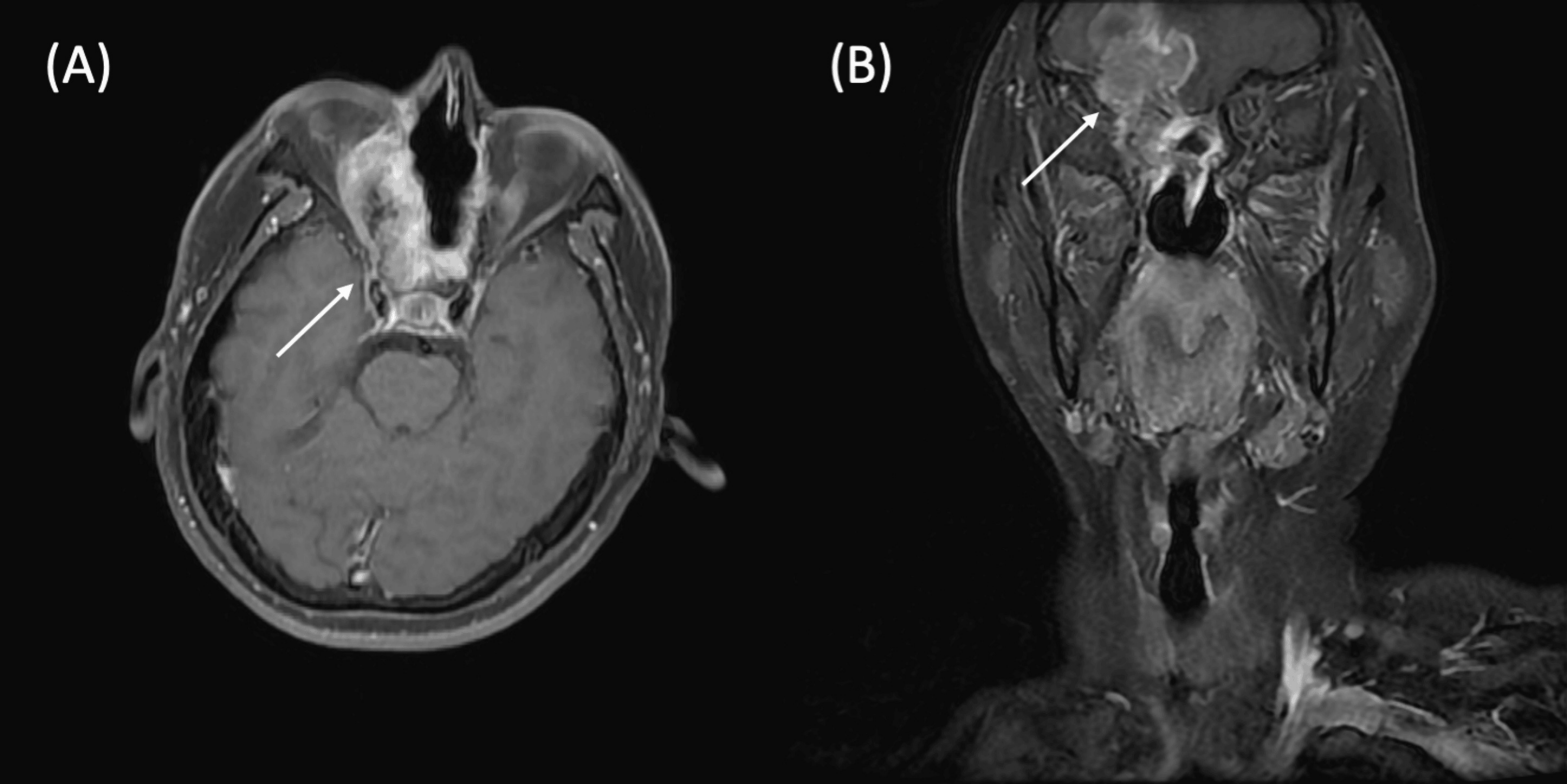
Axial and coronal view of contrast-enhanced T1 MRI at pre-treatment baseline. (A) Axial view and (B) Coronal view showing the pre-treatment baseline. White arrows indicate the location of the recurrent tumor mass.
